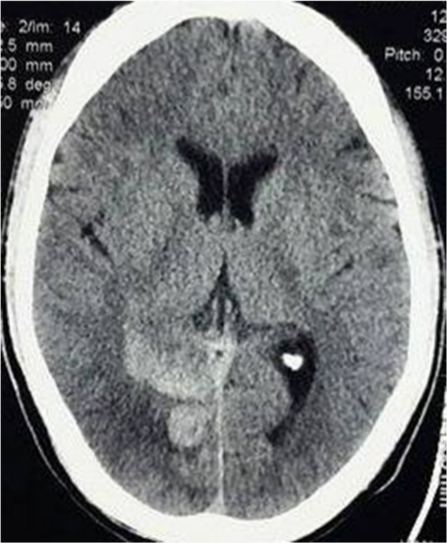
Axial non-contrast computed tomography of head showing grade II hemorrhagic transformation on the left occipital lobe
